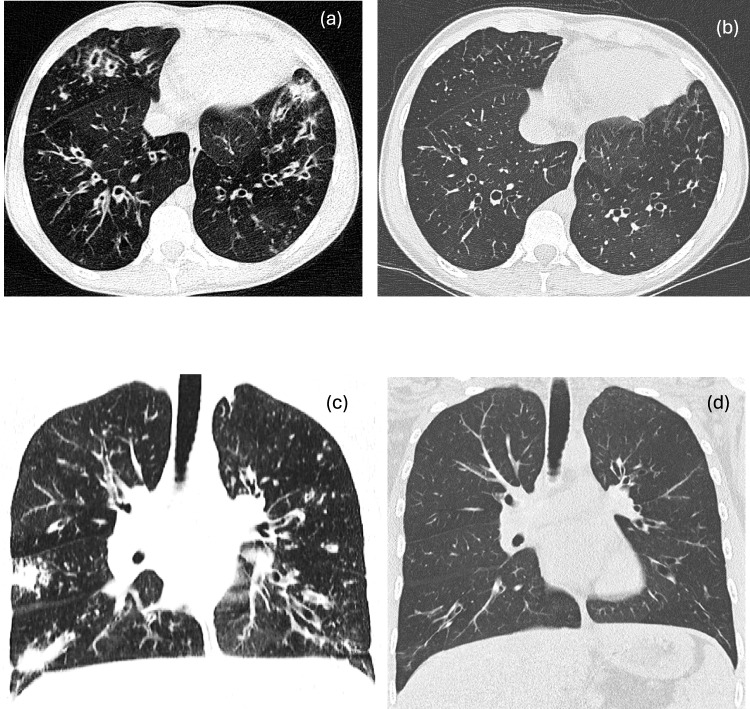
Axial (a, b) and coronal (c, d) representative CT-ULD images of 13-year-old female patient prior to initiation of therapy with Trikafta® and after 14 months. A reduction of bronchial wall thickening may be observed (a, b), consolidations disappeared (c, d)
